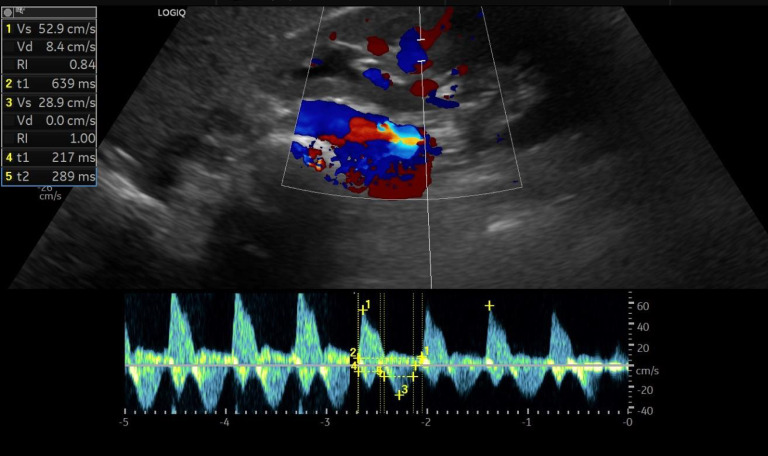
Intrarenale Dopplersonographie der rechten Niere. (Quelle: eigene Abbildung). In der Duplexsonographie Ableitung der A. interlobaris (oberhalb der Nulllinie) und V. interlobaris (unterhalb der Nulllinie). Relevante Messgrößen: IRVF-Muster biphasisch, VII = 1,0 und RVSI = 0,21. *IRVF* intrarenaler venöser Fluss, *RVSI* Renal Venous Stasis Index (Index der renalvenösen Stase), *VII* Venous Impedance Index (Index der venösen Impedanz)
